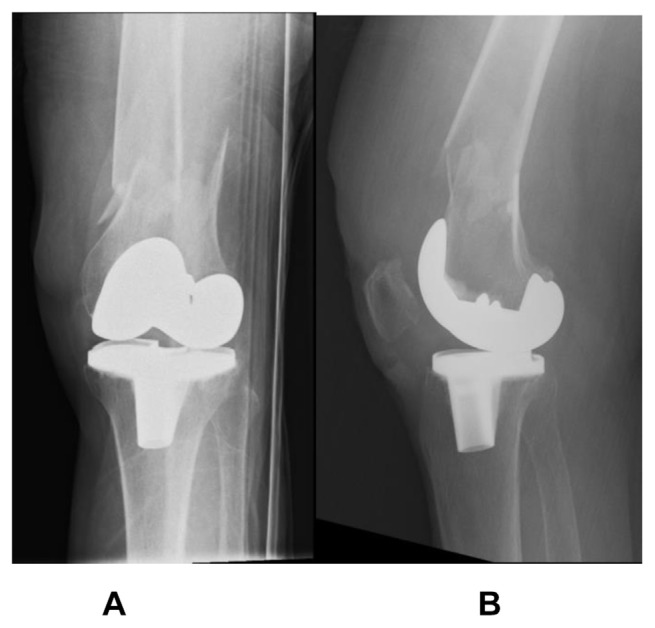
Preoperative radiographs show a periprosthetic fracture of the right distal femur.
